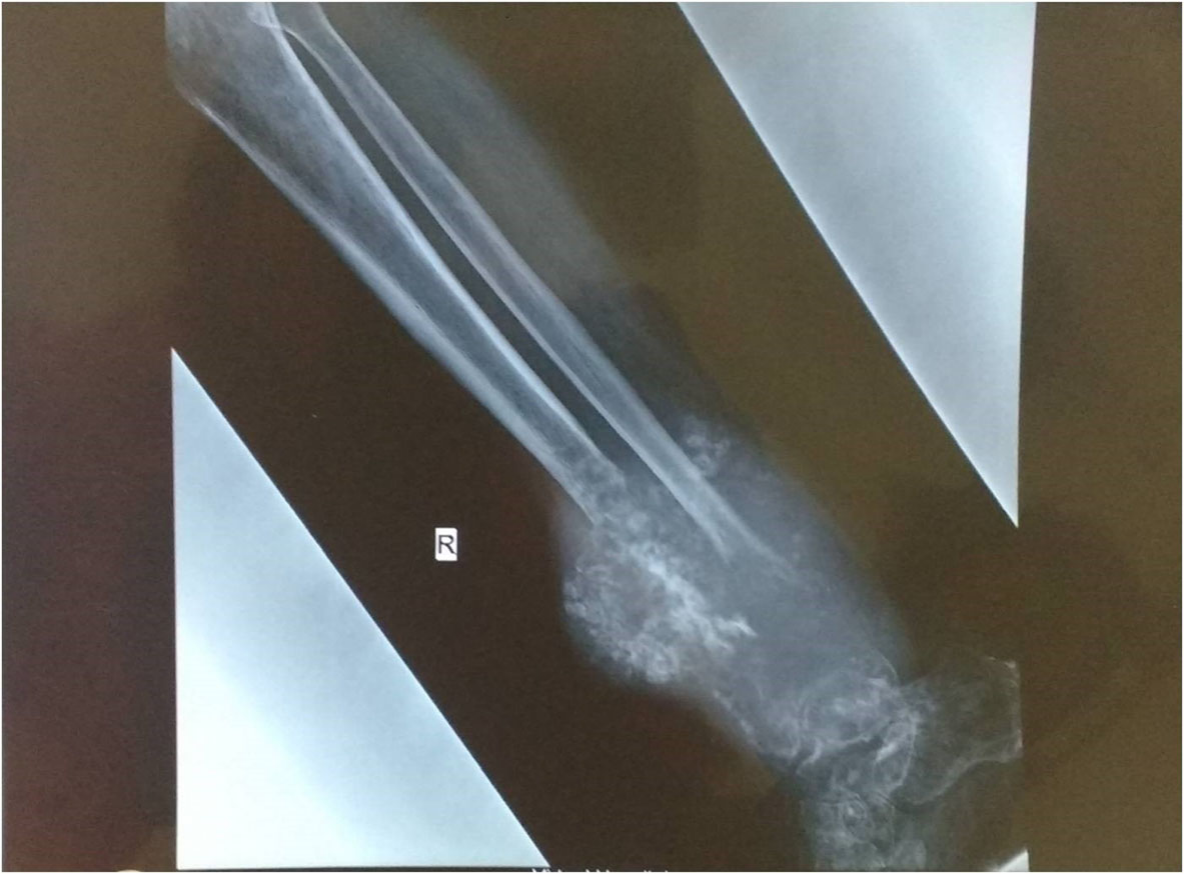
X-ray film of right leg showing large lytic expansile lesion involving middle and lower third of tibia and fibula
